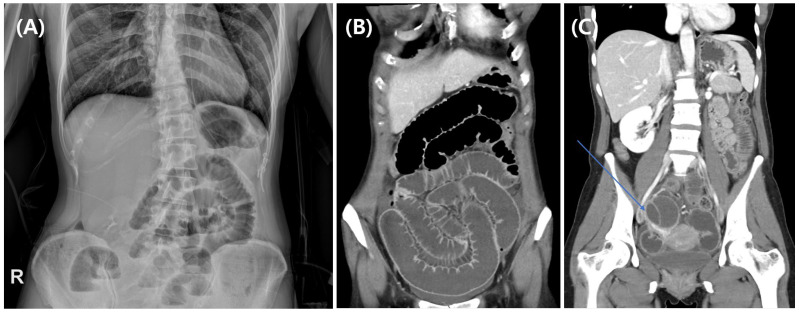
(A) Upright abdominal radiograph demonstrating dilated small bowel loops with multiple air–fluid levels. The “R” marker indicates the patient’s right side. (B) Coronal abdominal CT image showing dilated small bowel loops. (C) Axial abdominal CT image revealing a 5.5 × 5.0 cm right-sided ovarian cyst suggestive of endometrioma (blue arrow), with a transition point at the distal ileum in the pelvic cavity due to adhesion with the right adnexal cystic mass. Abbreviations: CT; computed tomography.
